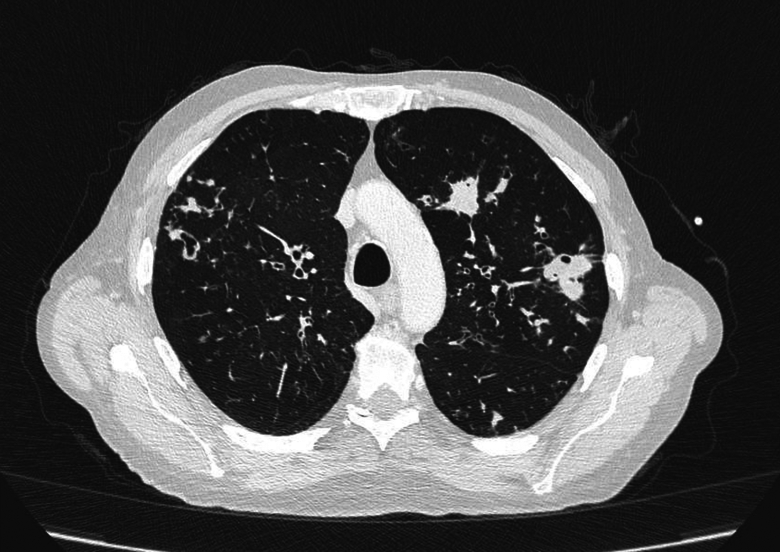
Chest CT scan demonstrates bilateral upper lobe bronchiectasis and a cavitation in the left upper lobe.
